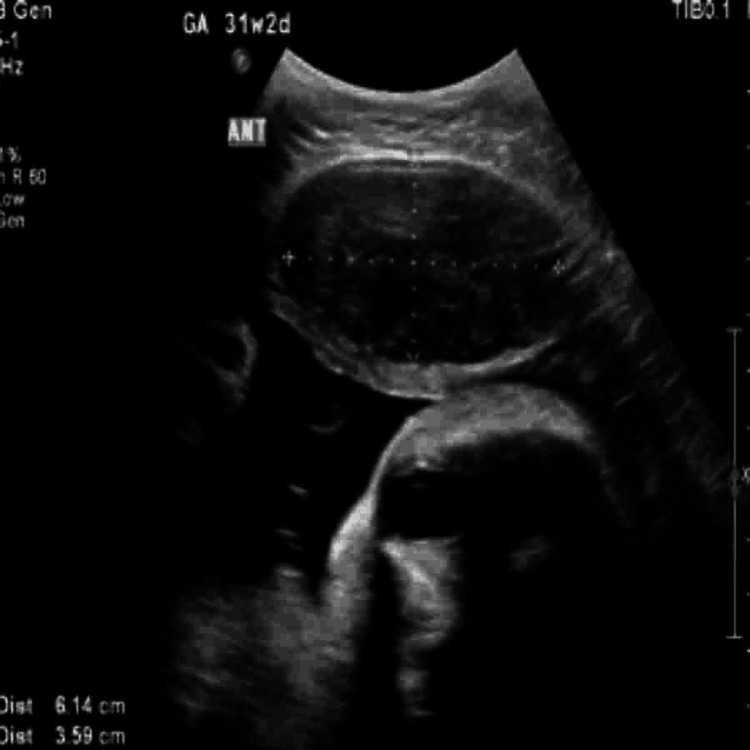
Ultrasound at 31 weeks and 2 days of gestation demonstrating a well-circumscribed hypoechoic anterior uterine wall fibroid measuring approximately 6.14×3.59 cm. The fibroid appears to compress the adjacent uterine structures without directly impinging on the fetus.
